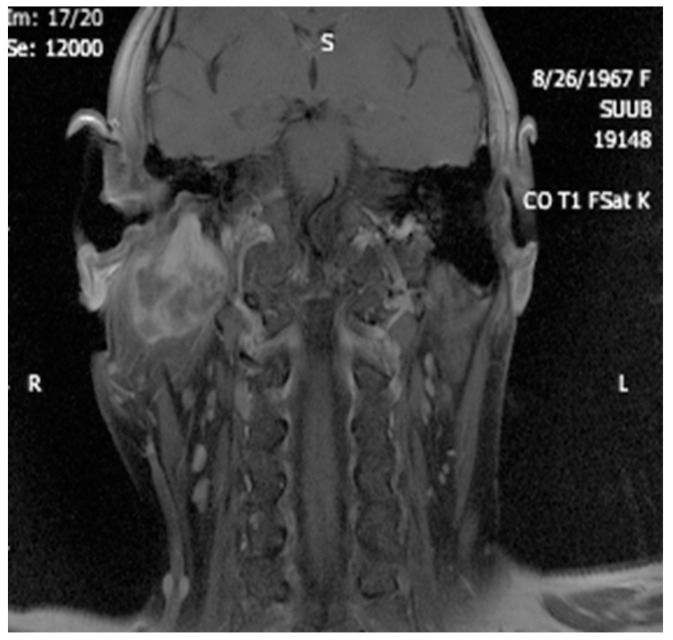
MRI appearance of a nonhomogeneous tumor developed at the level of the right stylomastoid foramen, with an appearance suggestive of a schwannoma of the right facial nerve (coronal section).
